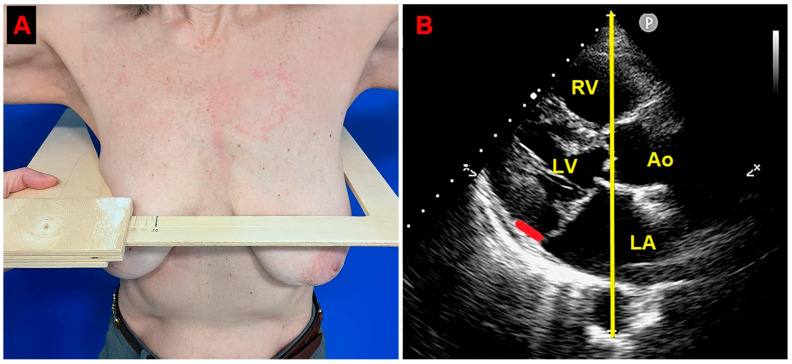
Modified Haller index, obtained by dividing the latero-lateral thoracic diameter by the anteroposterior thoracic diameter. (A) The latero-lateral thoracic diameter is measured by a rigid ruler coupled to a level. (B) The anteroposterior thoracic diameter is measured from the echocardiographic parasternal long-axis view as the distance between the true apex of the sector and the posterior wall of the descending aorta. The bold red line indicates the MAD distance. The bold yellow line indicates the anteroposterior thoracic diameter. Ao, aorta; LA; left atrium; LV, left ventricle; RV, right ventricle.
